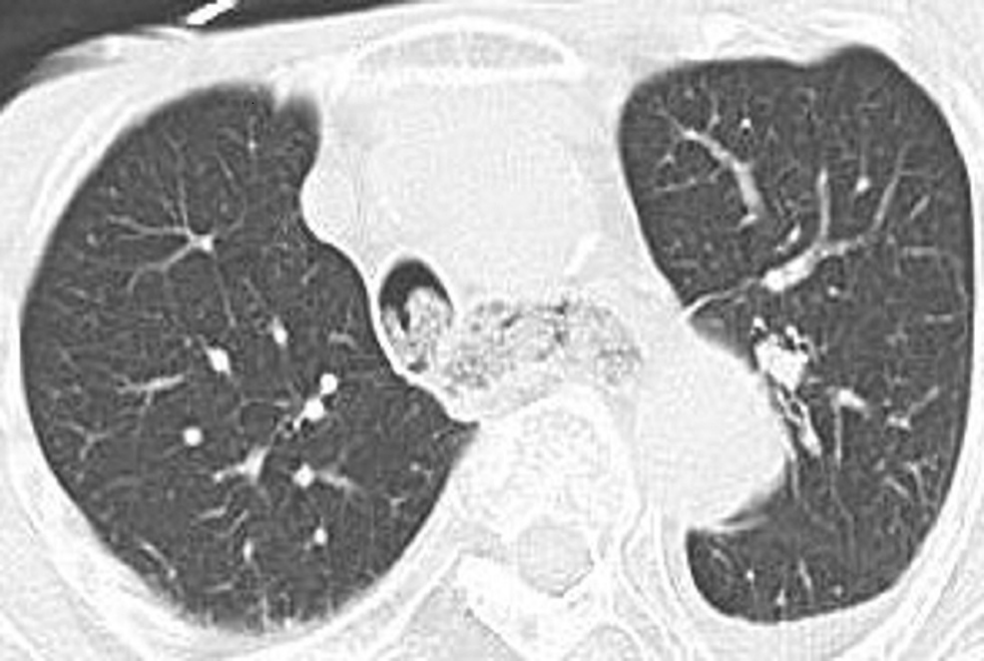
Foreign bodies filled in the trachea and esophagus on chest computed tomography
